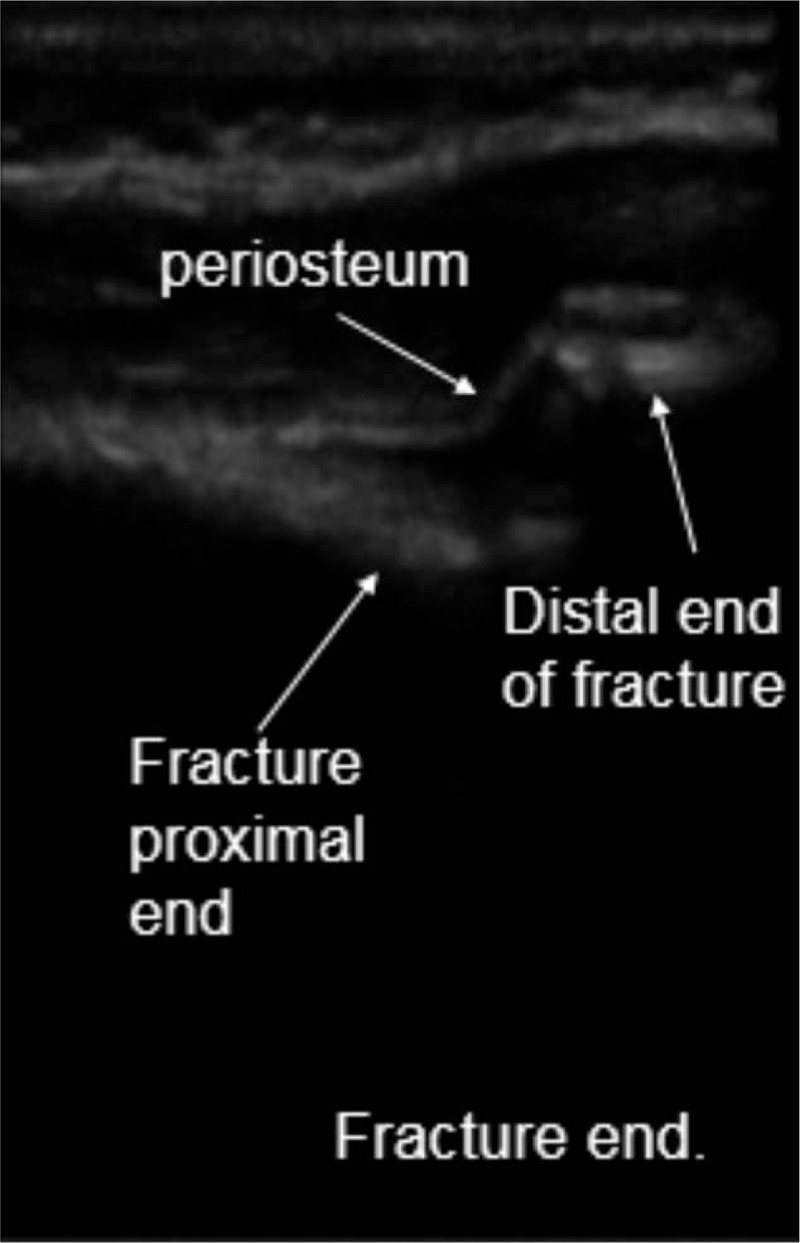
Intraoperative ultrasound to identify fracture sites.
